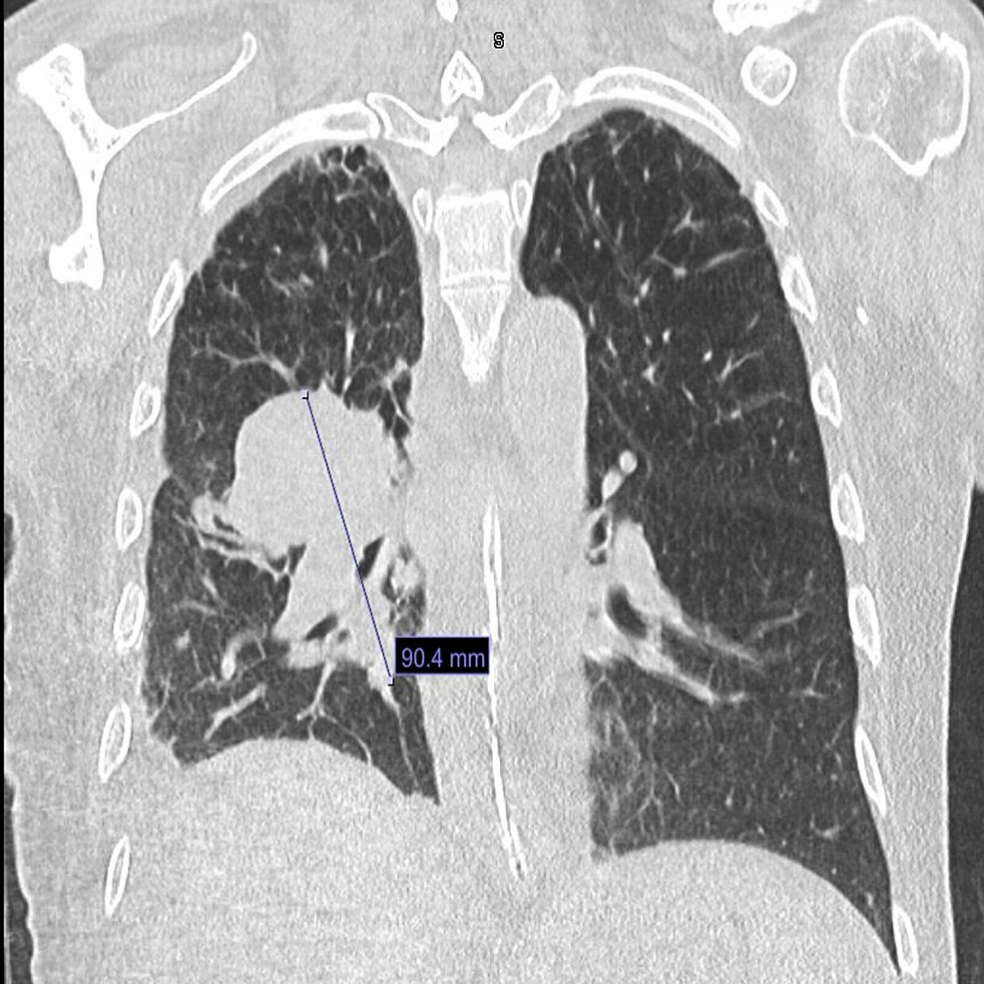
CT chest showing disease progression after immunotherapy with increasing mediastinal lymphadenopathy. CT: computed tomography
